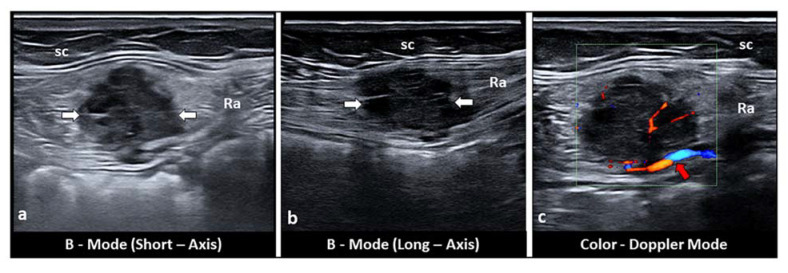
Short-axis (a) and long-axis (b) sonograms show a hypoechoic, nodular mass (white arrows), located inside the rectus abdominis (Ra) muscle. Of note, fine intralesional, vascular spots (red arrow) are depicted on the color Doppler mode (c). sc: subcutaneous tissue.
